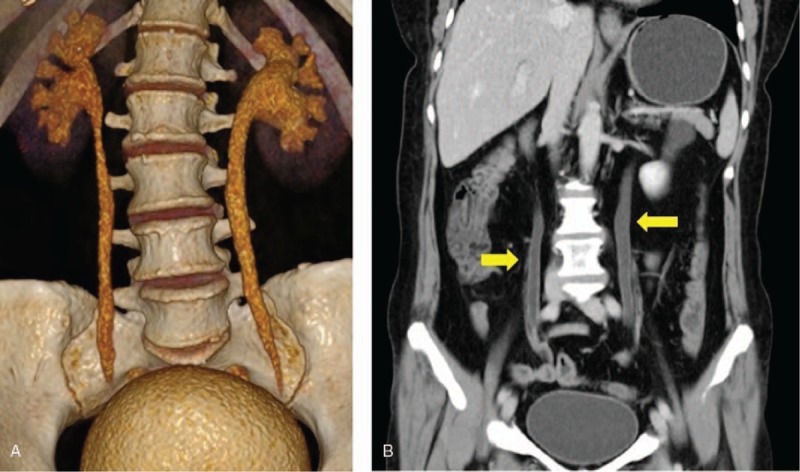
CT urography shows bilateral hydronephrosis, wall thickening of bilateral renal pelvis, and whole length of bilateral ureters with abnormal enhancement.
